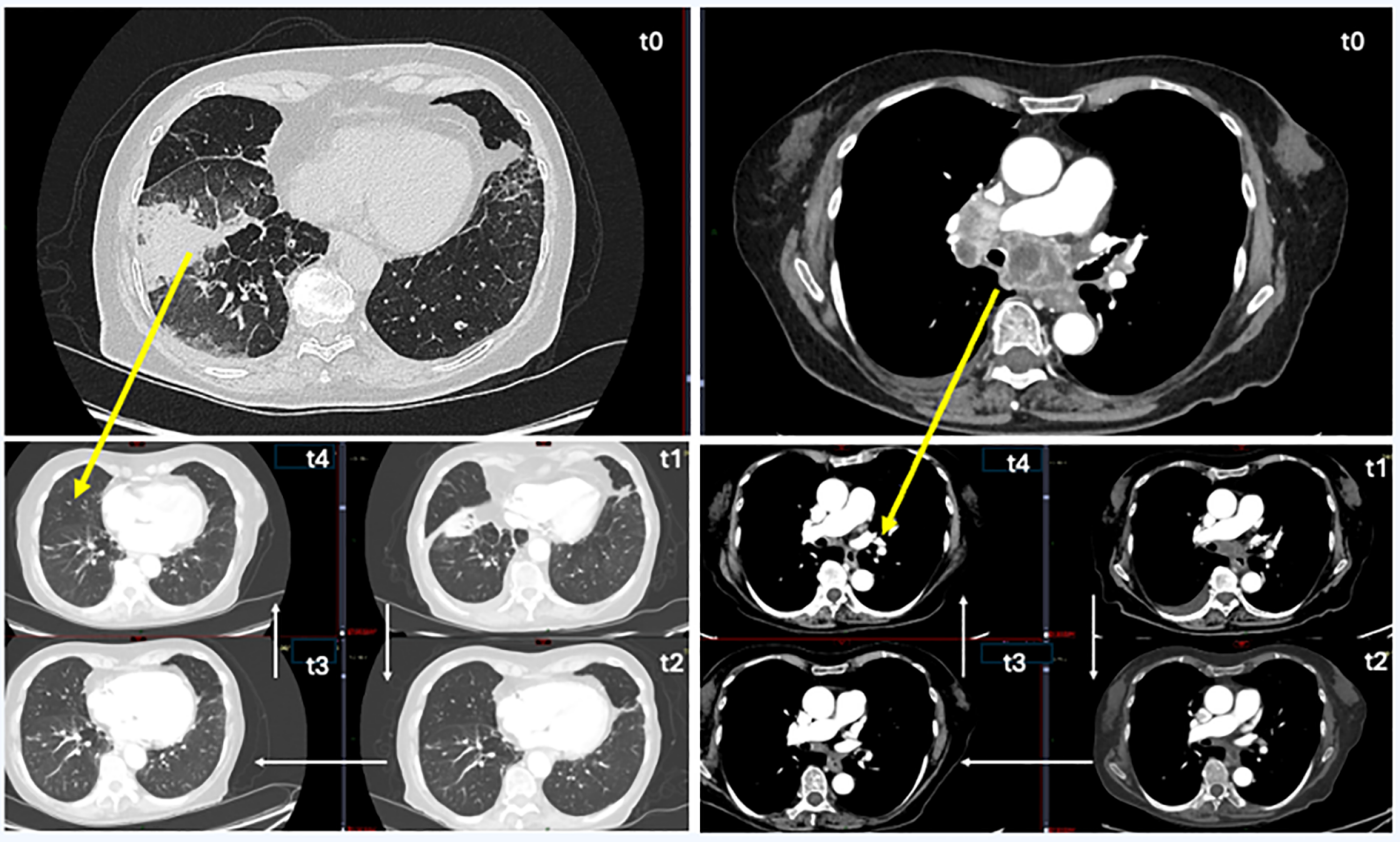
CT chest follow-up images. On the left, images with lung-window CT view, and on the right, images with mediastinal window. T0 (time 0): before therapy, pulmonary neoplasia in the right lung (lower lung lobe) and mediastinal subcarinal lymphatic localizations. T1 (after 3 months): start the reduction of lung parenchymal neoplastic mass and subcarinal lymphatic localizations. T2 (after 5 months): unrecognizable lung parenchymal neoplasm, further reduction of lymphatic localizations and their contact with the airways and pulmonary arteries. T3 (after 9 months): unrecognizable lung parenchymal neoplasm, marked reduction of subcarinal lymphatic localizations. T4 (after 12 months): no radiological evidence of oncological disease, optimal response to treatment.
